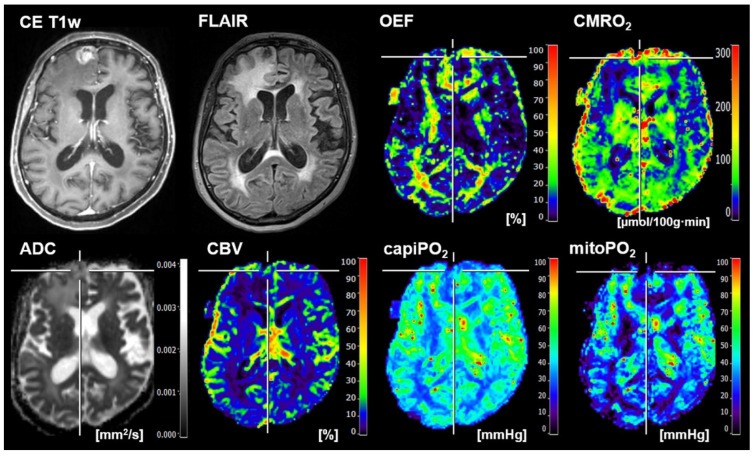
Representative case of a 66-year-old female patient suffering from a brain metastasis from melanoma that was misclassified as GB by the 1D-CNN but correctly classified by the radiologists. Contrast-enhanced (CE) T1w and FLAIR MRI data combined with the quantitative maps of apparent diffusion coefficient (ADC) and cerebral blood volume (CBV) among others were used for human reading by two board-certified radiologists in consensus. MRI biomarker maps for oxygen metabolism including oxygen extraction fraction (OEF), the cerebral metabolic rate of oxygen (CMRO_2_), capillary oxygen tension (capiPO_2_), and mitochondrial oxygen tension (mitoPO_2_), respectively, of the patient, are presented on the right-hand part of the Figure.
